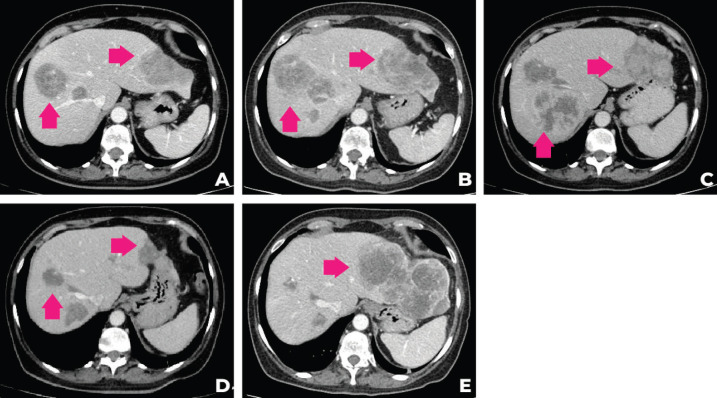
Evolution of the patient’s computer tomography scans in different time periods. (a): CT of December 2020, (b): April 2021, (c): June 2021, (d): September 2021, (e):December 2021.
